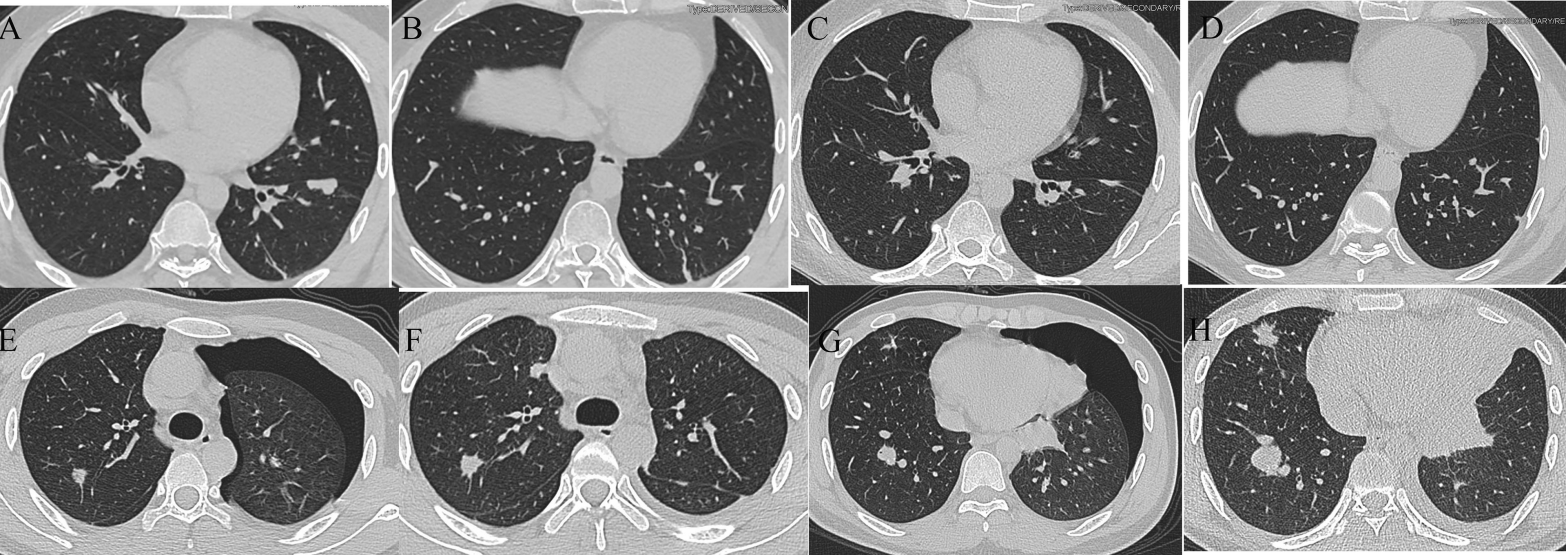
Lung CT results of one PD and one DCR patient. (A, B) Lung CT results of a DCR patient before immunotherapy; (C, D) Lung CT results of a DCR patient after immunotherapy; (E, F) Lung CT results of a PD patient before immunotherapy; (G, H) Lung CT results of a PD patient after immunotherapy.
